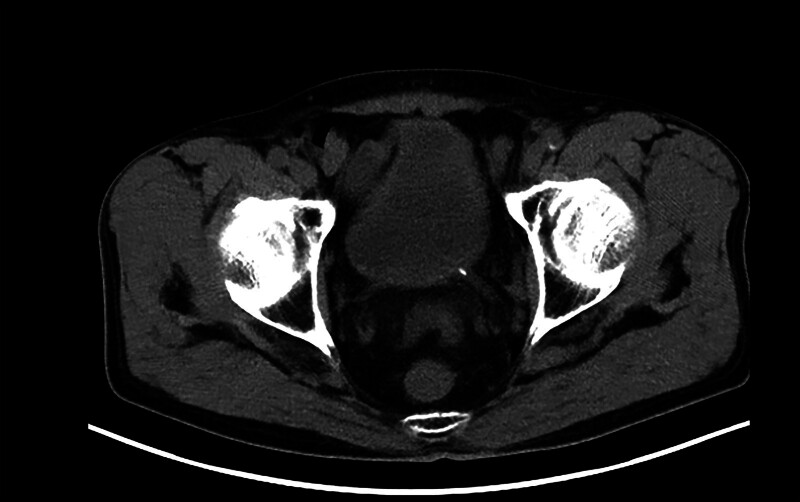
A CT scan of the urinary tract revealed a left ureteral stone measuring approximately 6 mm × 4 mm. CT = computed tomography.
